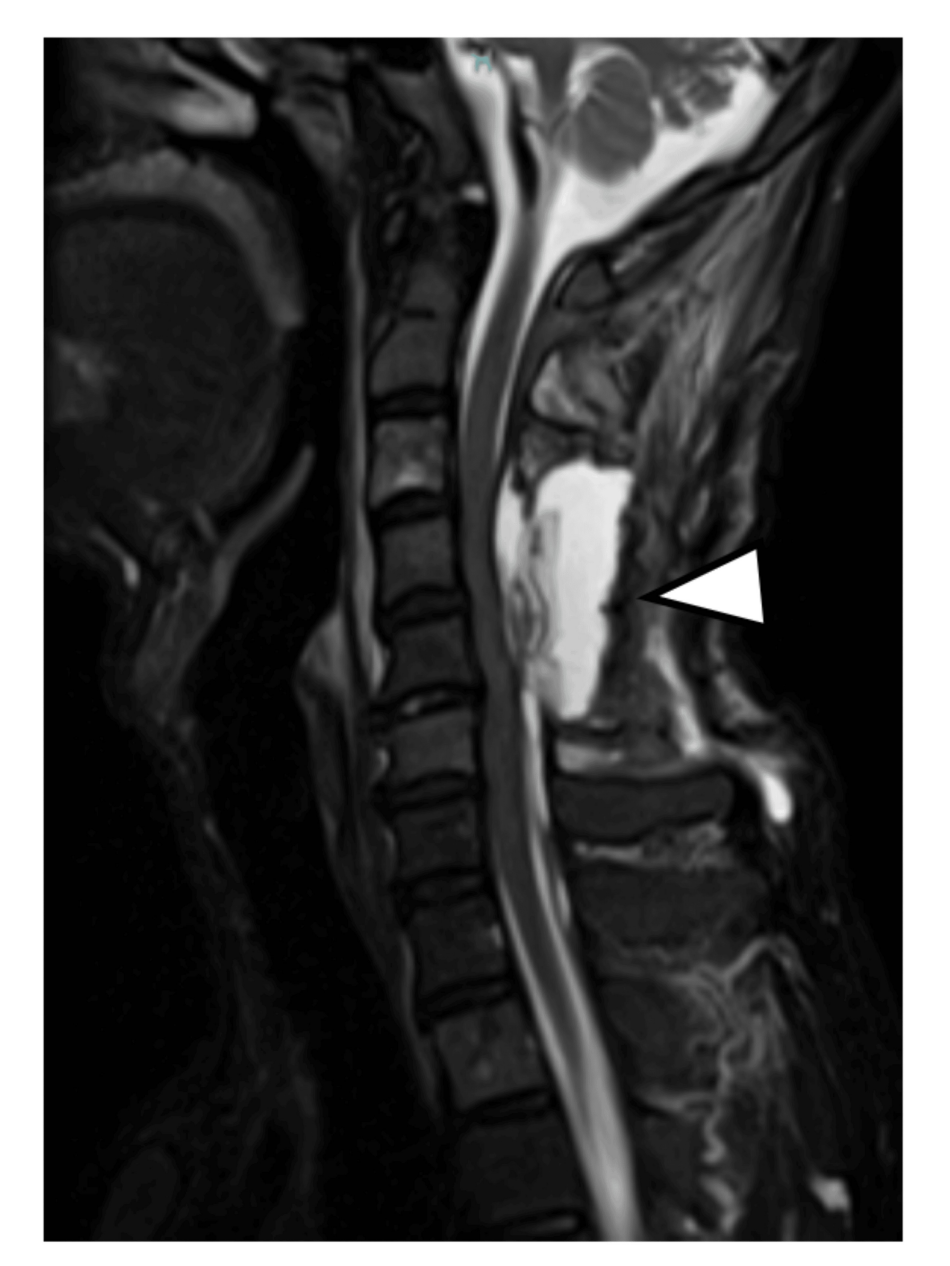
Cervical spine MRI (T2-weighted sagittal image) obtained 20 days after operation Recurrent retained effusion and cervical cord compression were observed (arrowhead) MRI: magnetic resonance imaging
